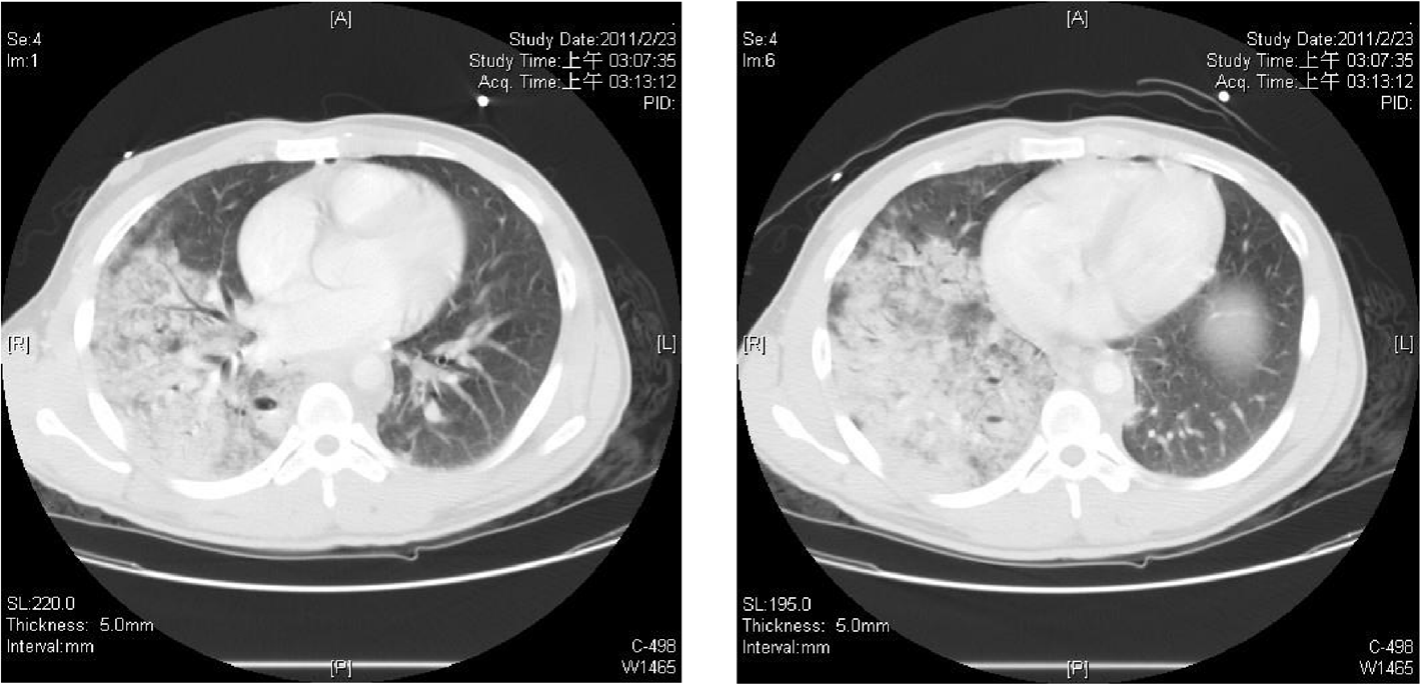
Chest CT showed right lung consolidation with patchy opacities.
